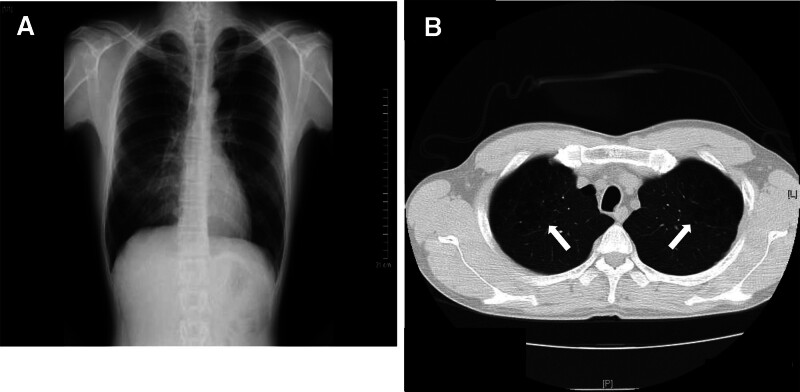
CR and LDCT images of COPD case taken on the same day. (A) CR reveals no abnormal opacity. (B) LDCT shows a number of low-attenuation areas in the bilateral upper lung fields (white arrows). COPD = chronic obstructive pulmonary disease, CR = chest radiography, LDCT = low-dose computed tomography.
